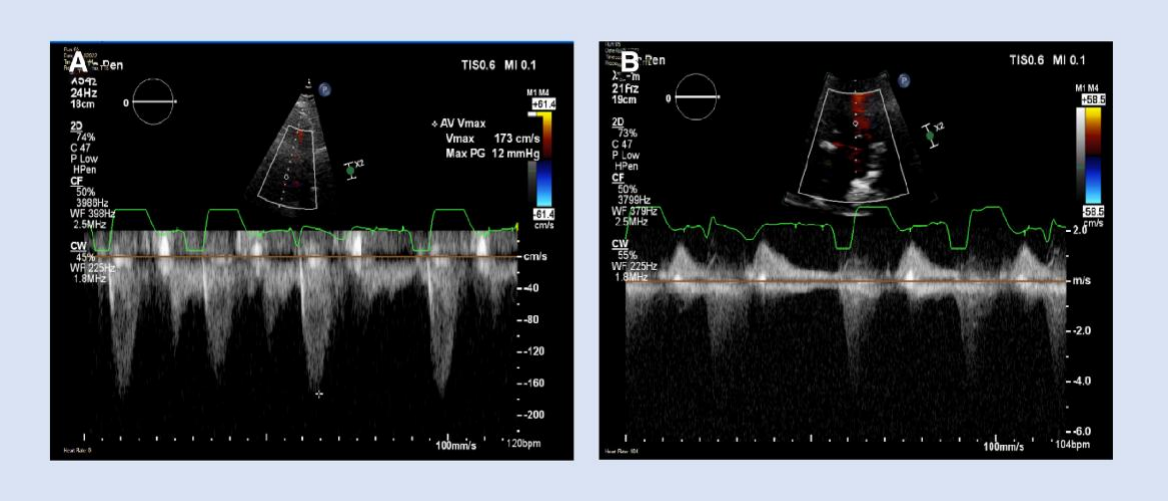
(*A*) Continuous-wave Doppler (CWD) across the aortic valve in the A5C view demonstrating no haemodynamically significant obstruction by the cardiac mass. (*B*) CWD in the A2C view demonstrating mild mitral regurgitation jet.
